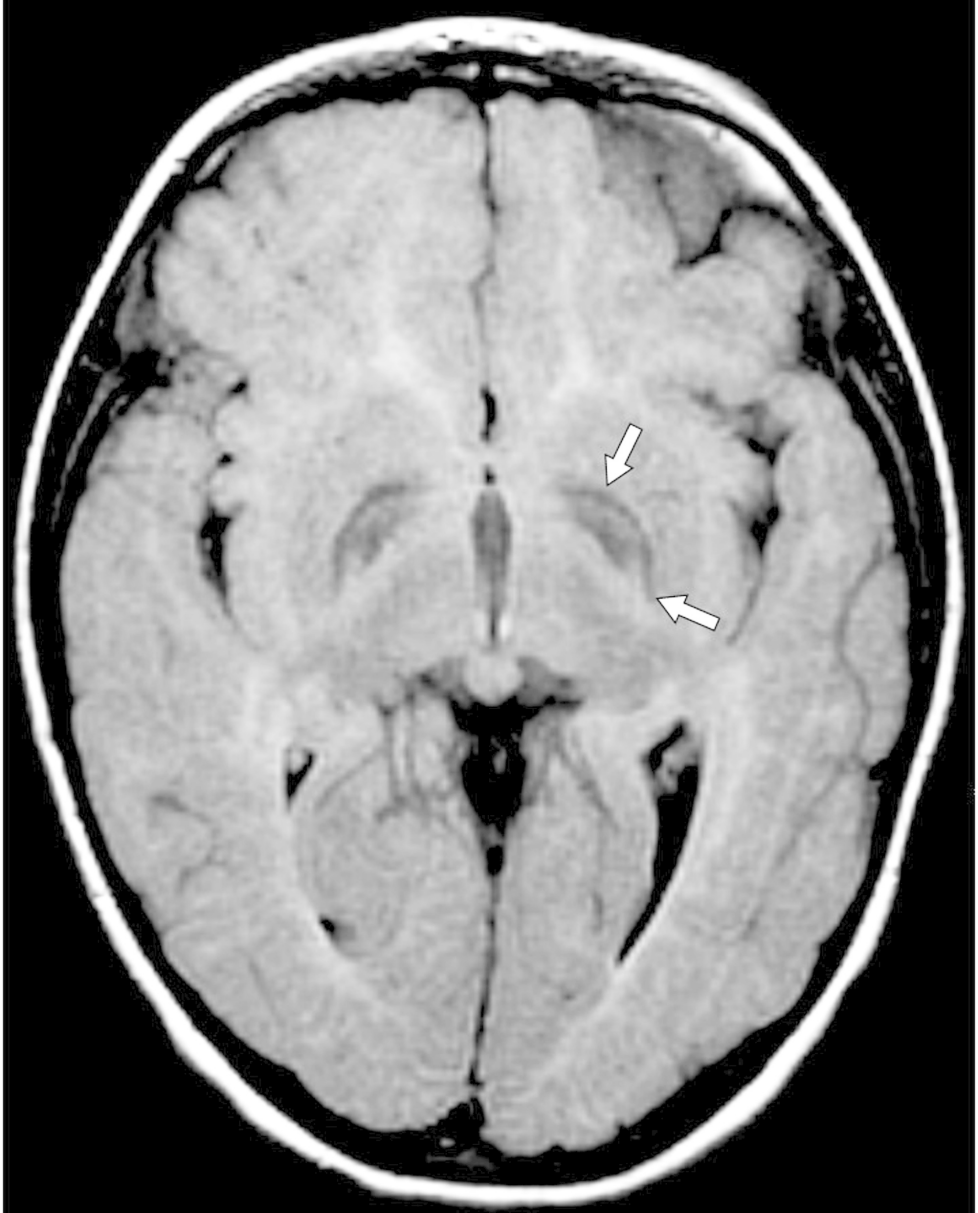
Globi pallidi showing symmetrical high T1 and low T2/ T2-fluid-attenuated inversion recovery (FLAIR) signal with characteristic curvilinear bands (horizontal plane)
